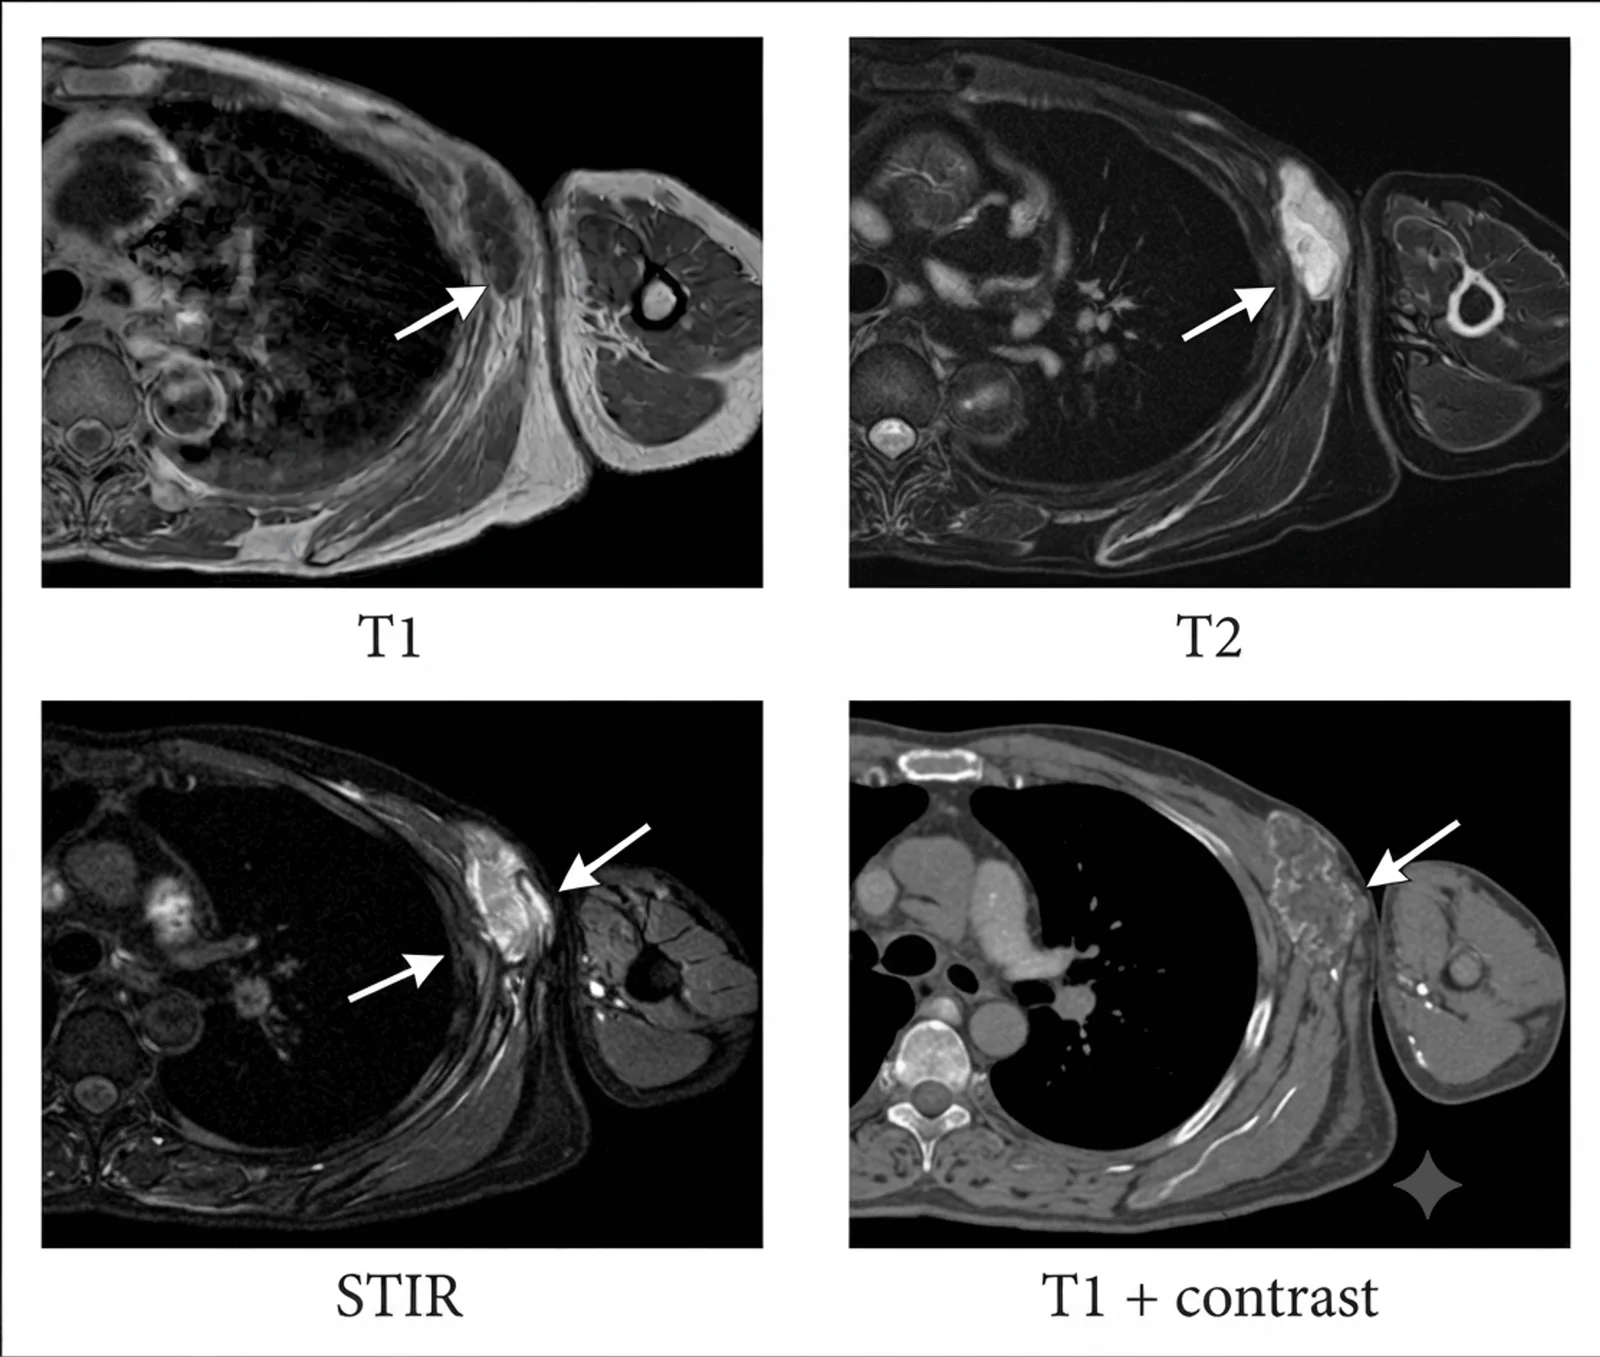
Axial MRI sequences of the left axilla demonstrating the left axillary/chest wall lesion. Axial MRI sequences presented in a four-panel grid: T1-weighted (T1), T2-weighted (T2), STIR, and post-contrast T1-weighted (T1+C) sequences. The white arrows indicate a mass located in the left axillary/chest wall, which appears heterogeneously hyperintense on T2 and STIR images and shows heterogeneous enhancement after gadolinium administration. MRI, magnetic resonance imaging; STIR, short tau inversion recovery
